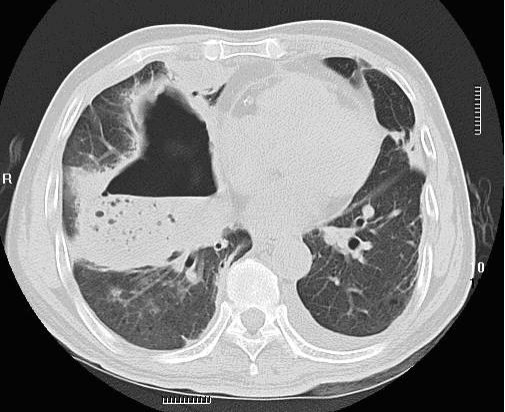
Computed tomography scan of the thorax. The scan shows features typical of pulmonary abscesses, consolidation with a single cavity containing an air-fluid level in the right lung after six days of intravenous cefepime treatment.
